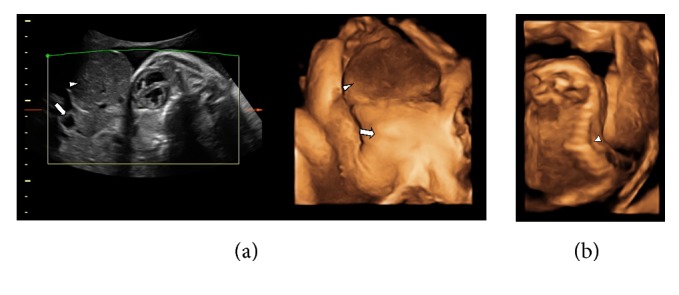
(a) 2D and 3D ultrasound image showing abdominal wall defect with liver (short arrow) and small bowel (long arrow) herniation at 33 weeks of gestation. (b) 3D ultrasound image showing kyphosis of the thoracolumbar spines at 22 weeks of gestation (arrow).
